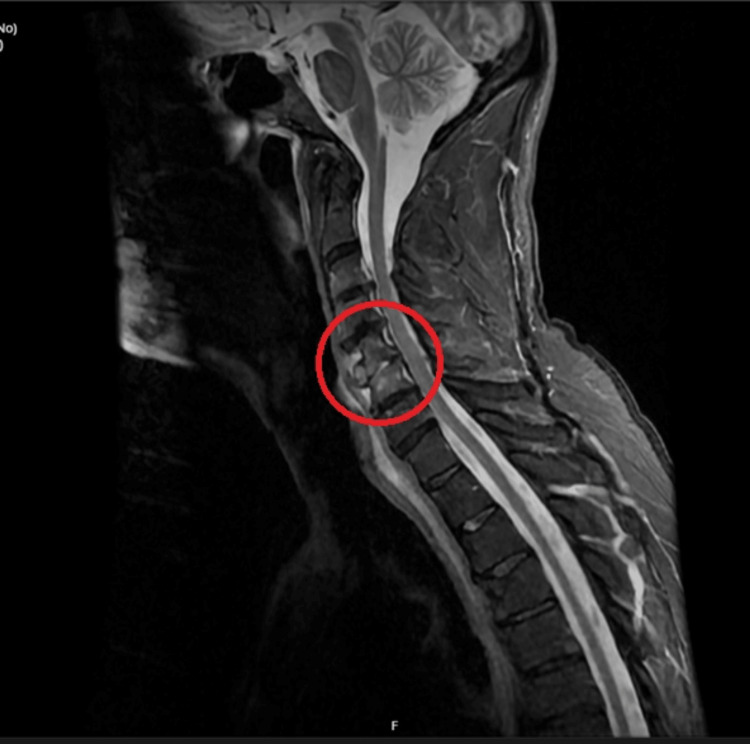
MRI showing discitis at the level of vertebrae C4 & C5. The red circle demarcates the area of discitis.
